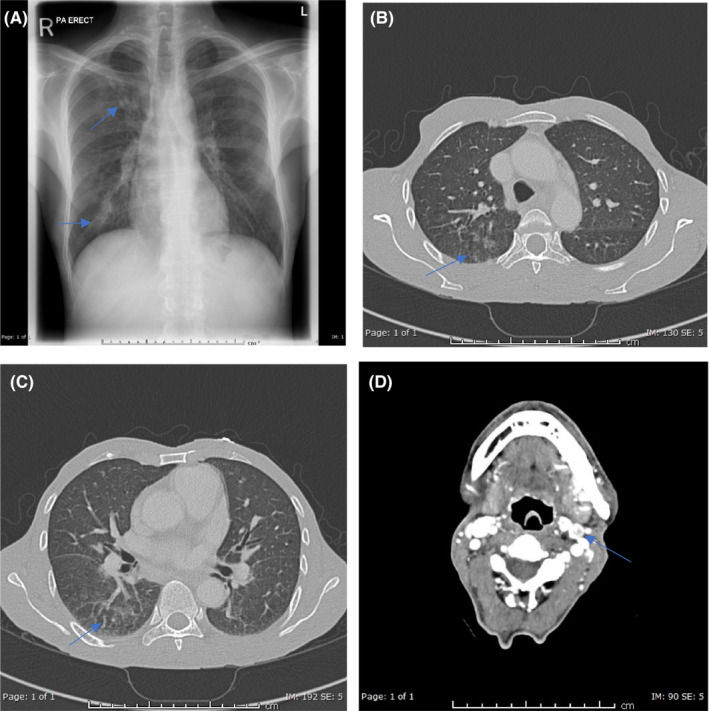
A, Chest X‐ray (PA view) showing small patchy areas of airspace shadowing in the right lung base and in the right infraclavicular region to a lesser extent (arrows). B, C, Axial CT of the chest lung window showing small patchy areas of ground‐glass opacities and nodular infiltration in the posterior segment of the right lung upper lobe (arrow in B) and in the apical segment of the right lower lobe (arrow in C). D, Axial postcontrast study of the neck showing bilateral cervical subcentimeter‐enhancing lymph nodes, some of which show central nonenhancing areas likely representing necrosis (arrow)
